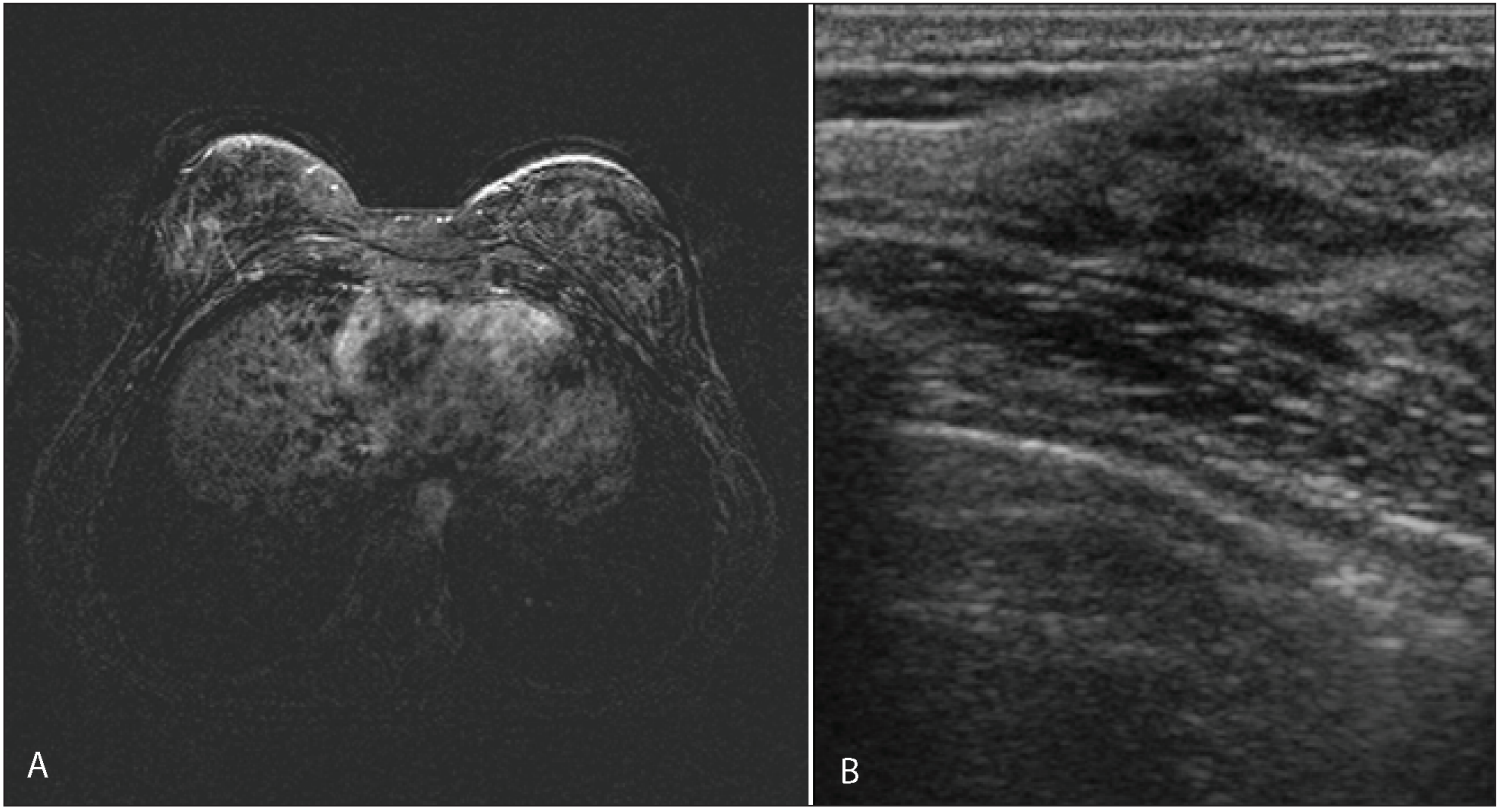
2014: MRI of the breasts, T1 substraction image after contrast administration shows a marked decrease in contrast enhancement (A). On ultrasound the volume and acoustic shadowing of the lesion has also decreased (B).
